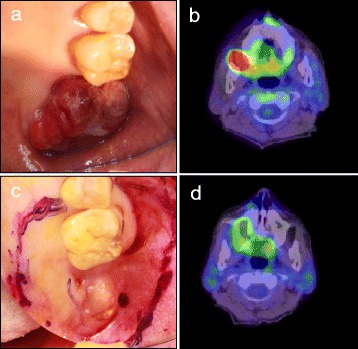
Clinical photographs and imaging data. Clinical photographs (a, c) and positron emission tomography/computed tomography (PET/CT) imaging (b, d) of the lesion. Initial presentation (a, b), 40 days after biopsy when surgery was performed (c), and 102 days after biopsy (d). The exophytic tumor had clinically disappeared
